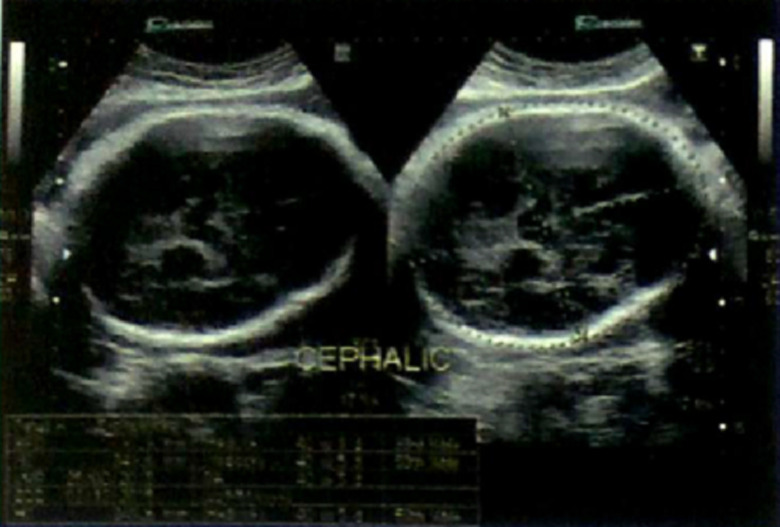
antenatal ultrasound scan of brain
